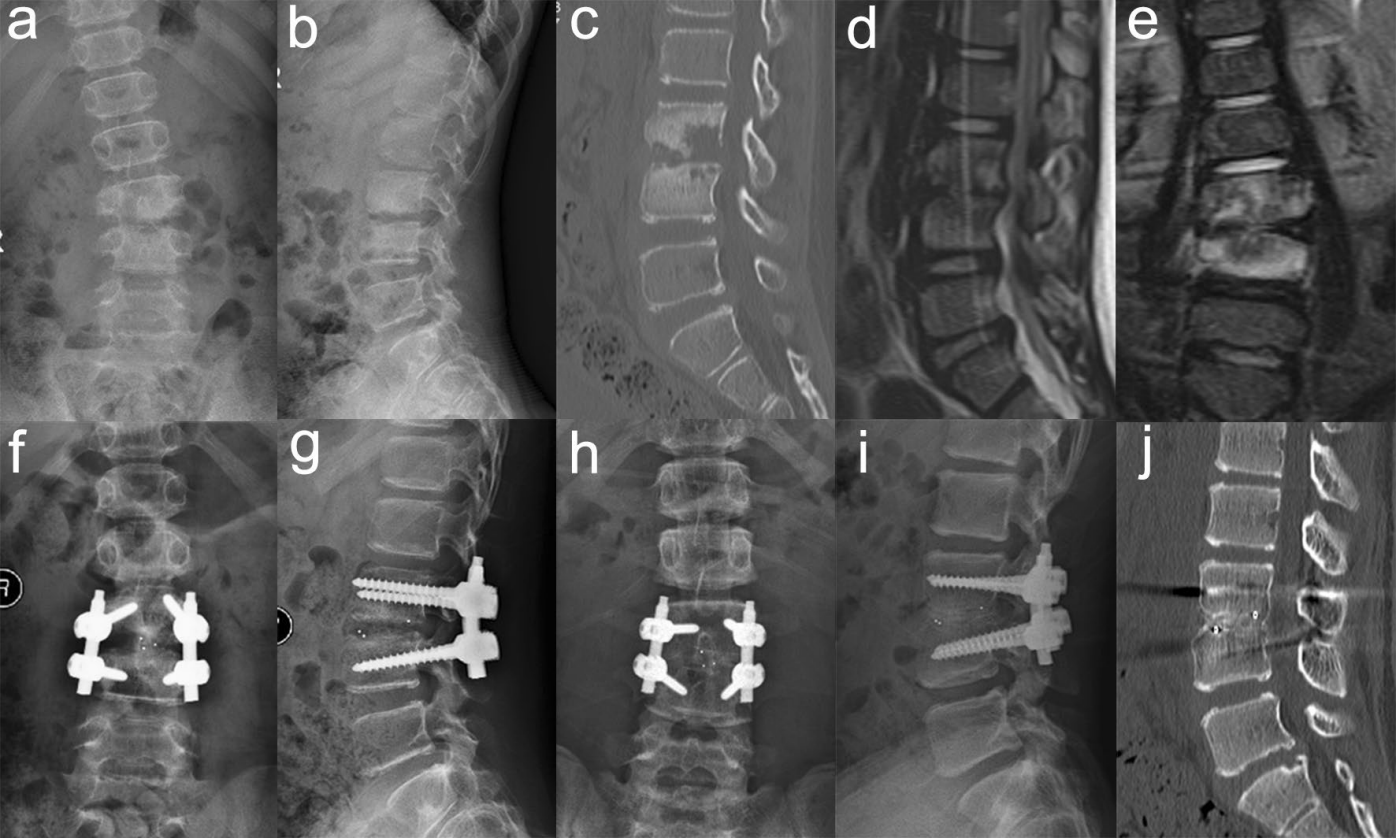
A 12-year-old boy with spinal tuberculosis in L3 to L4 underwent posterior-only approach using PEEK cage combined with single-segment instrumentation. (a–e) Preoperative images showing the collapse of vertebral body and imbalanced spinal growth. (f–g) Postoperative radiograph revealing good internal fixation and correction of imbalanced spine. (h–j) 49-month follow-up images depicting internal fixation in a good position and solid bone fusion.
